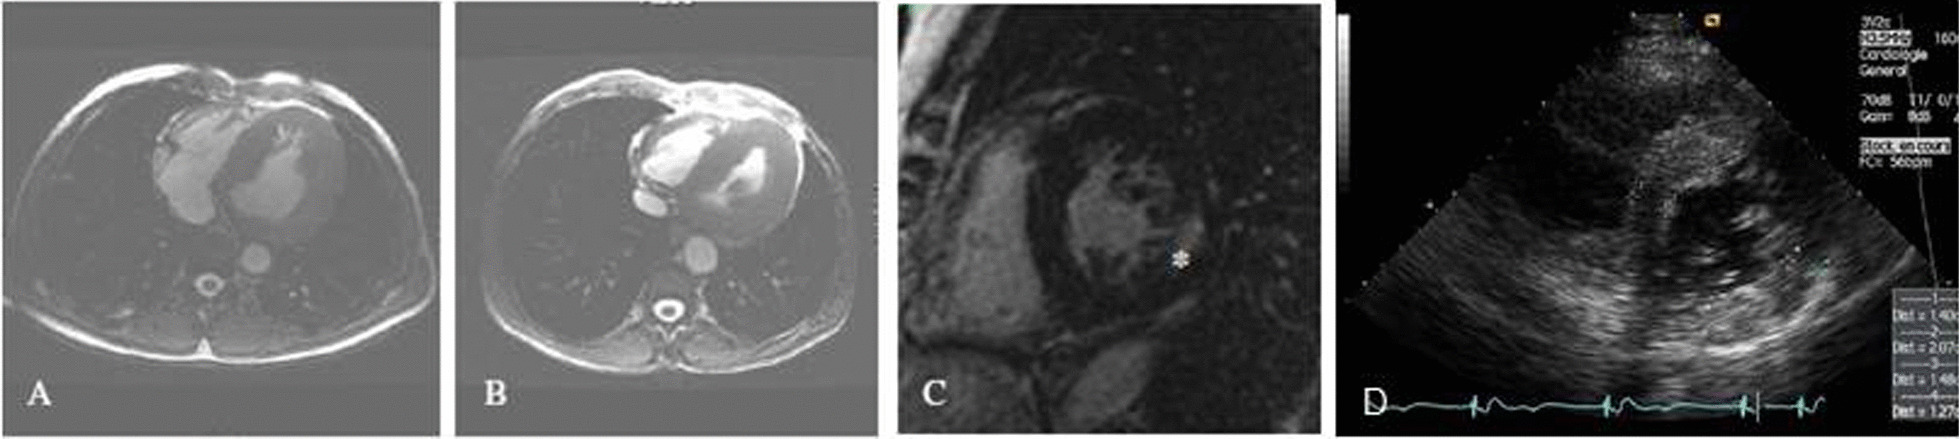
Cardiac MRI for the assessment of left ventricular hypertrophy and fibrosis. A Left ventricular hypertrophy in a 51-year-old male patient with cerebrovascular involvement and end stage renal disease (dialysis). B Hypertrophic cardiomyopathy in a 56-year-old male patient with arrythmya, leukoareiosis and kidney transplant. C Late enhancement after gadolinium in a 63-years-old female patient with end stage renal disease (dialysis). D Echocardiography: parasternal short axis showing left ventricular hypertrophy. Adapted from Germain Orphanet Journal of Rare diseases 2010, 5:30
